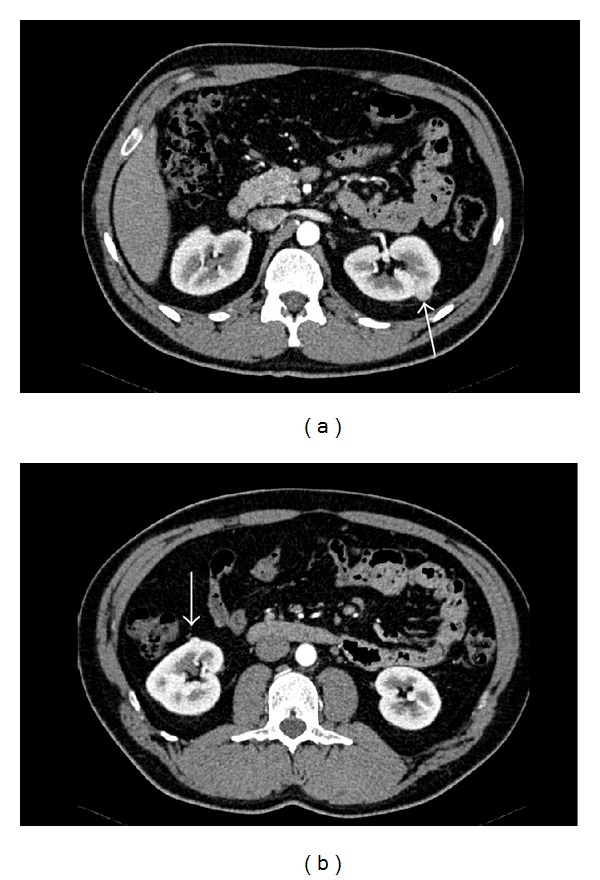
Contrast enhanced CT shows in the arterial phase a hypervascular small lesion in both kidneys, representing two small chromophobe renal cell carcinomas. The tumour in the left kidney (a) was treated by radiofrequency ablation (RFA); for the tumour in the right kidney, (b) follow up was proposed.
